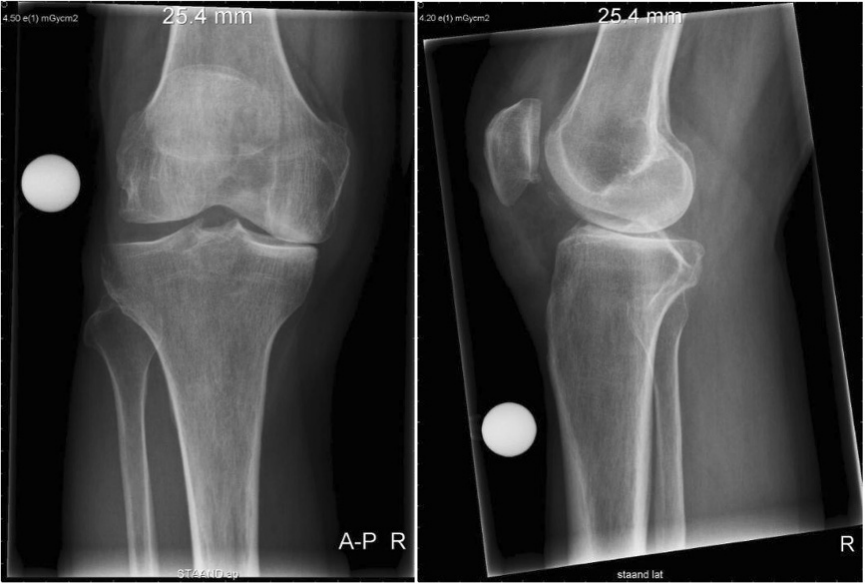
Preoperative anterior-posterior and lateral X-rays showing anteromedial osteoarthritis of the right knee.
